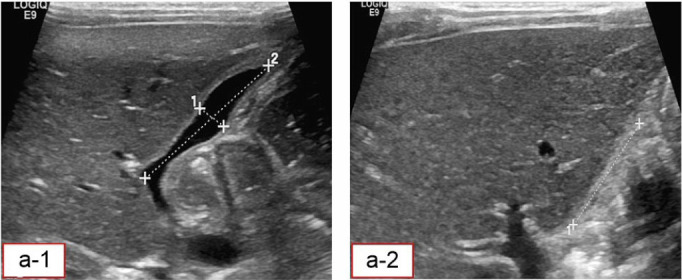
Ultrasound image of a normal GB with normal contraction. a-1, GB length (2) and width (1) after 10 hours fasting. a-2, GB length after drinking a bottle of milk, and depicts the collapsed width.
